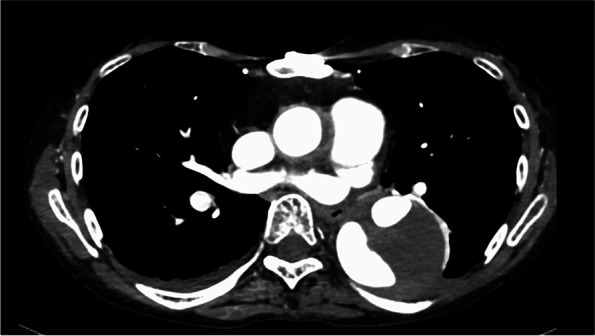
Computed tomography image 9 days before surgery
